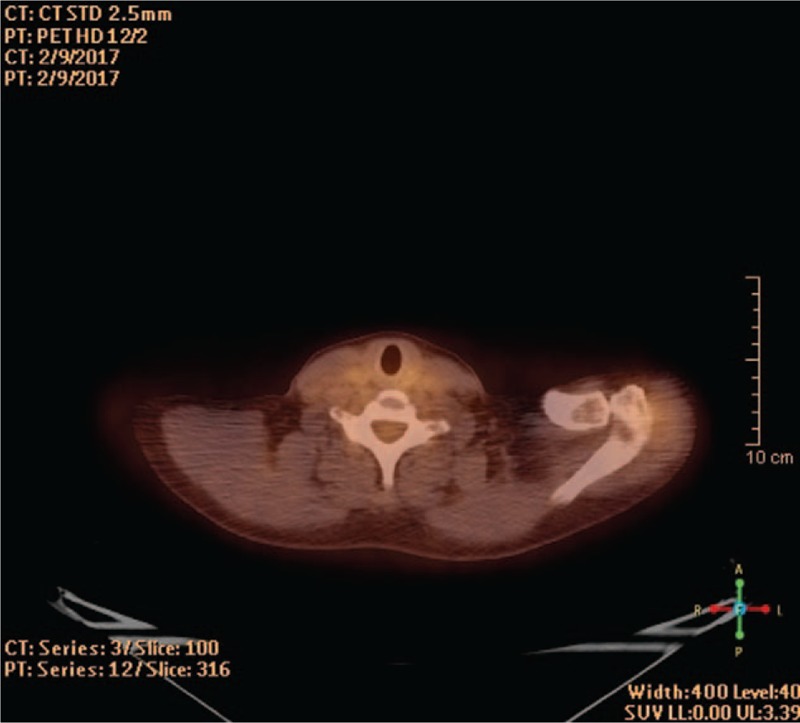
Follow-up FDG-PET/CT scan—enlarged, but PET negative lymph nodes in the right cervical region (known to be iodine positive on post-treatment SPECT CT) consistent with DTC lymph node metastases.
